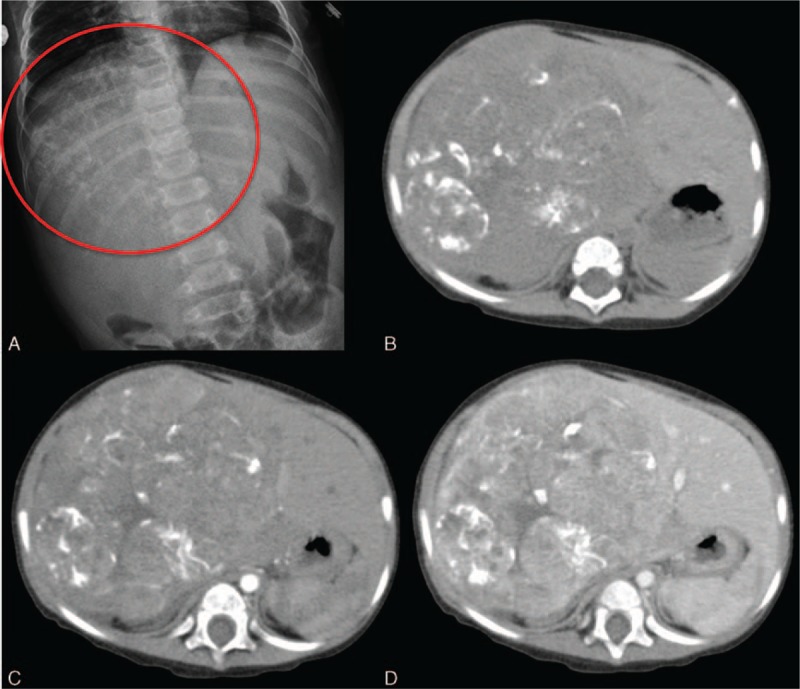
A 1-year-old girl with a mixed type hepatobastoma. A, Amorphous calcification (circle) is noted at the right upper abdomen on the plain radiograph. This characteristic should raise the suspicion of hepatoblastoma in the infant. B, Calcification within the tumor masses indicates osteoid, cartilaginous, or fibrous components, usually seen in mixed-type hepatoblastoma. C, D, Subtle arterial enhancement without significant washout on the later phases (D). Some subtle enhancement in the septations on the delayed phase is depicted as well.
